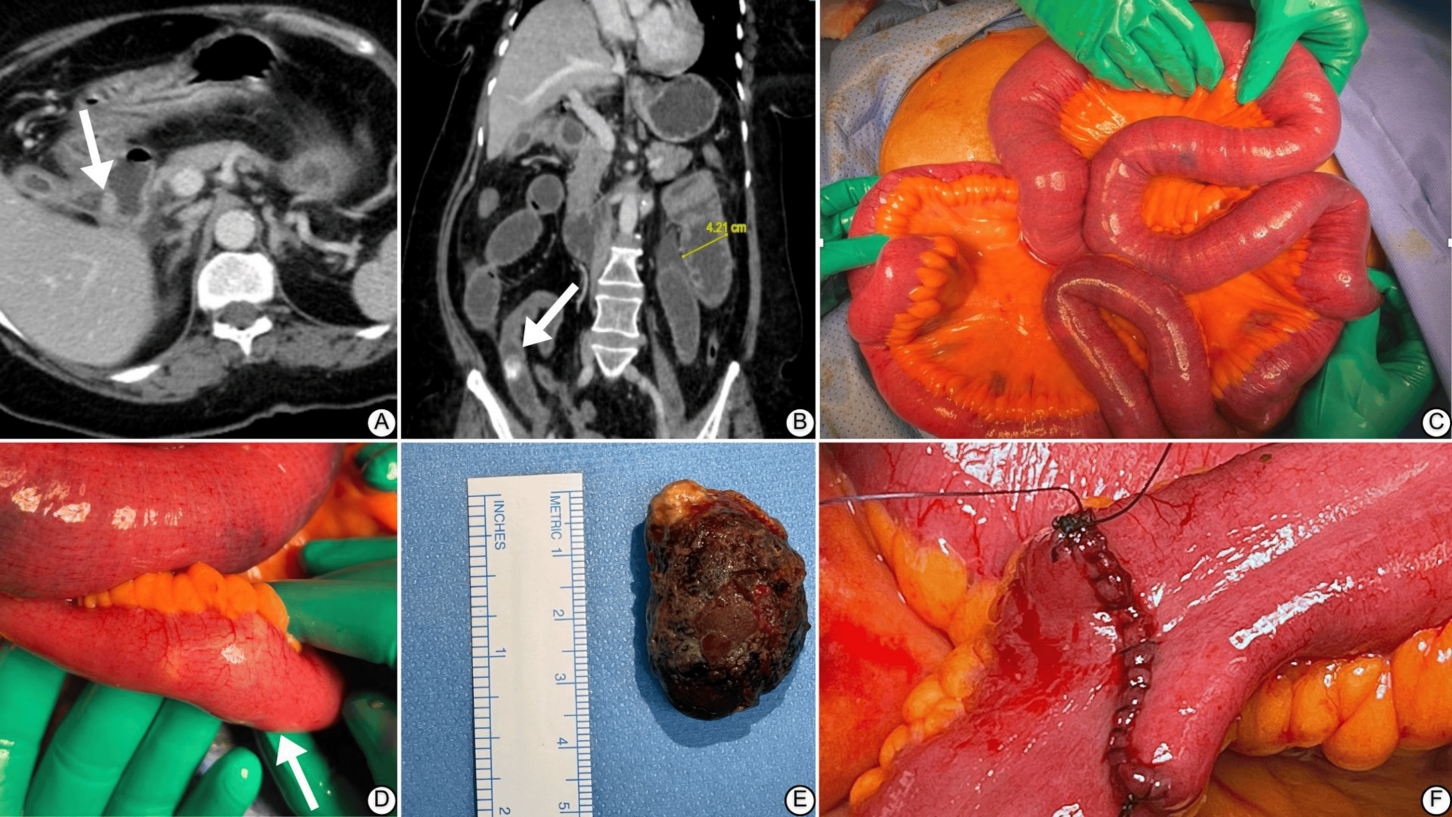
Gallstone ileus – CT images and intraoperative findings (A) The axial CT image shows a dilated gallbladder with an arrow pointing to the site where the gallbladder lumen communicates with the first part of the duodenum. The thickened walls of both the gallbladder and duodenum, along with surrounding fat stranding and free air, are indicative of chronic inflammation and the formation of a biliary-enteric fistula. (B) The coronal CT image highlights a 4.21 cm dilation (yellow line) and the stone (marked by an arrow). The distended loops of the small intestine proximal to the obstruction are visible, along with reactive bowel wall thickening. (C) The surgical field is exposed, showing dilated loops of the small intestine. the small bowel is significantly distended as a result of obstruction. (D) A closer view of the obstructed segment of the small intestine shows a bulge (arrow) where gallstone is identified. The impacted gallstone caused a complete blockage of the bowel. The bowel wall appears thickened and inflamed around the site of the obstruction. (E) The removed gallstone measures approximately 3.5 cm in size, shown next to a ruler for scale, with an irregular, rough surface. (F) The final step of the surgery shows the enterotomy site being closed transversely with continuous 3-0 Vicryl sutures. The closure is reinforced with Lembert continuous sutures using the same material, ensuring a secure and leak-proof seal.
